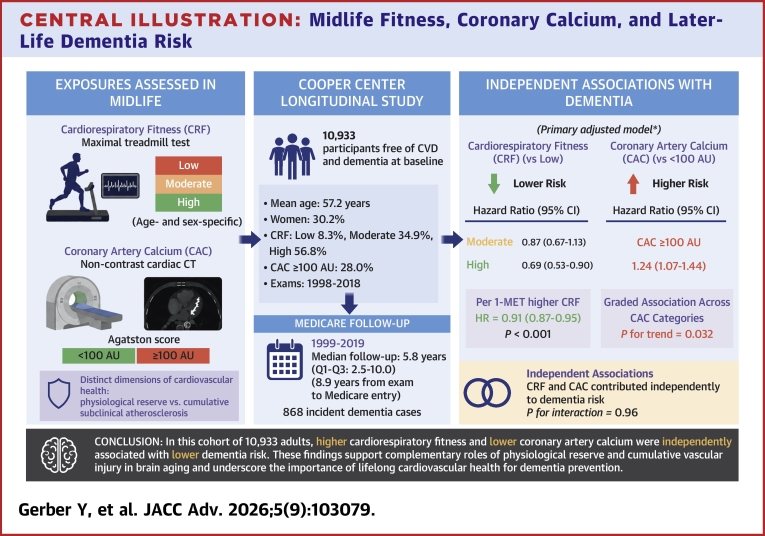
Midlife Fitness, Coronary Calcium, and Later-Life Dementia Risk Among 10,933 adults free of cardiovascular disease and dementia at baseline, cardiorespiratory fitness (CRF) and coronary artery calcium (CAC) were assessed in midlife and related to subsequent dementia identified through Medicare surveillance. Higher CRF was associated with lower dementia risk, whereas higher CAC burden was associated with greater risk. Associations remained after adjustment for individual, behavioral, and cardiometabolic risk factors and were consistent in categorical and continuous analyses. No evidence of interaction was observed, indicating that CRF and CAC contribute independently to dementia risk. These findings support complementary roles of physiological reserve and cumulative vascular injury in brain aging and underscore the importance of lifelong cardiovascular health for dementia prevention. ∗Primary adjusted model mutually adjusts for both CRF and CAC and includes: age, sex, year of exam, body mass index, smoking status, education, fasting glucose, total cholesterol, and systolic blood pressure (baseline hazards stratified by sex). AU = Agatston units; CVD = cardiovascular disease; CT = computed tomography; MET = metabolic equivalent of task.
